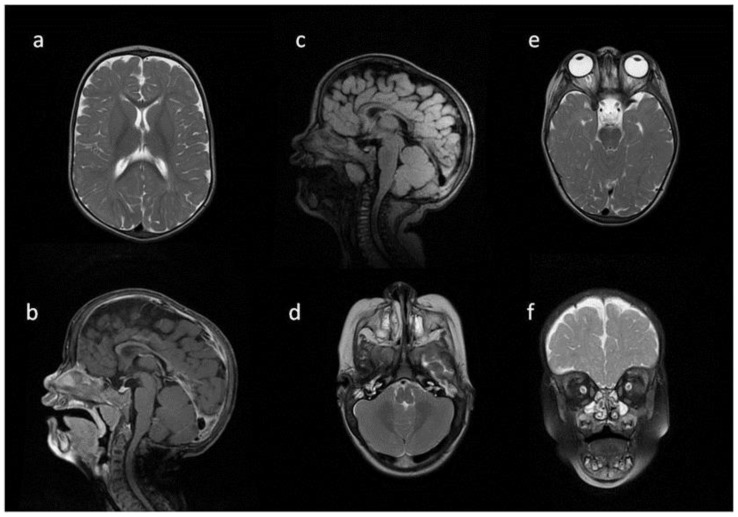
Brain MRI. Patient 3. (a,e,d) T2 axial; (f) T2 coronal; (b,c) T2 sagittal; (b) T1 contrast-enhanced; (c) T2 FLAIR. Findings: (a) delayed myelination; (b,c) J-shaped sella turcica, dysplastic dens; (d) temporal bone air cells effusion, posterior fossa horns; (e,f) enlarged optic nerve sheath.
